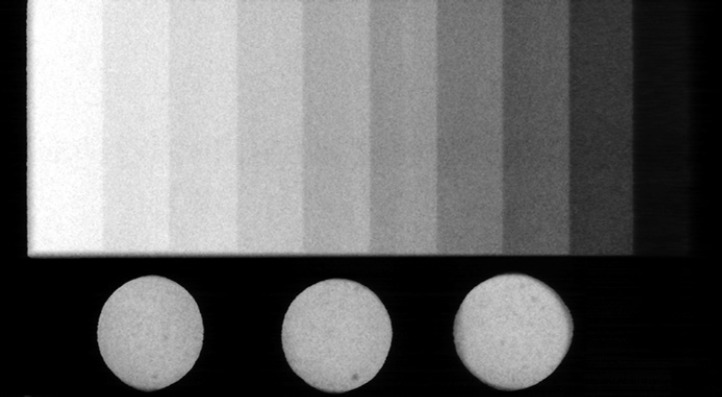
Digital radiographic image of HealApex with an aluminum step-wedge graduated from 0.5 to 5 mm Al (in 0.5-mm increments
